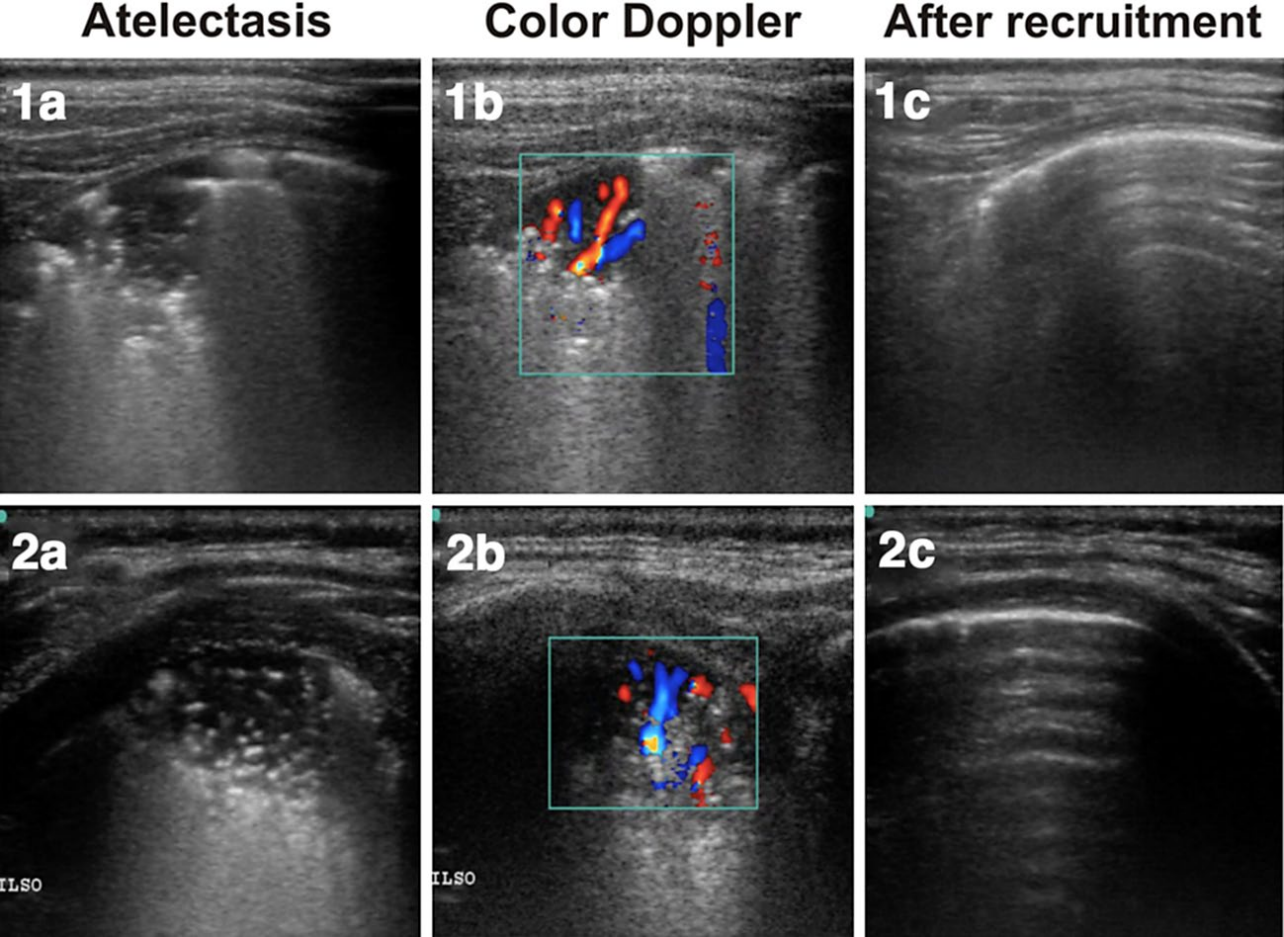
Color Doppler images of intra-pulmonary shunt. The images belong to two mechanically ventilated anesthetized children: (1) 1 year old and (2) 2 months old. a Atelectasis is observed as subpleural consolidations with air bronchograms and coalescent B-lines. b Color Doppler revealed lung vessels within the atelectasis showing a radial distribution to the lung periphery. c Normal lung tissue after successful treatment of the atelectasis by a recruitment maneuver, the normal aeration prevents the pulmonary vessels from being seen by lung sonography. These images correspond to the Additional file 1: Video 1
